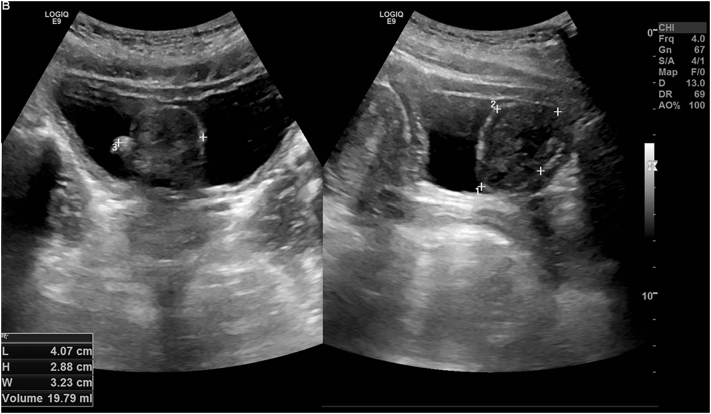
Ultrasound of the kidneys, ureters, and bladder revealing a prominent polypoidal lesion from the bladder mucosa with well-defined, encapsulated margins containing a small appendicular lobule on the surface.
